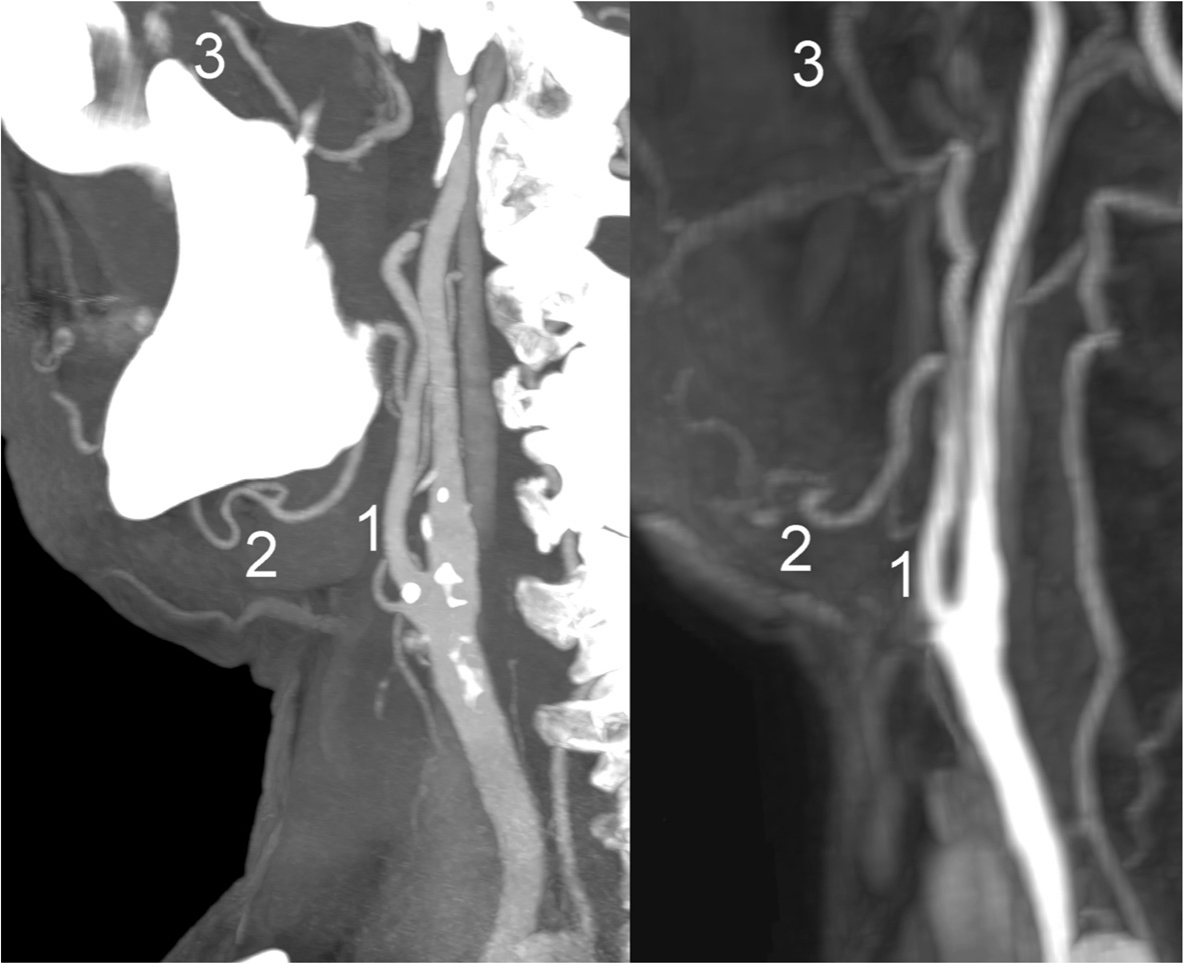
Sagittal maximum intensity projection (MIP) reconstructions of computed tomography angiography (left) and subtracted magnetic resonance angiography (right) images of the cervical vasculature of a patient scheduled for microvascular reconstruction. 1: external carotid artery; 2: facial artery; 3: maxillary artery.
